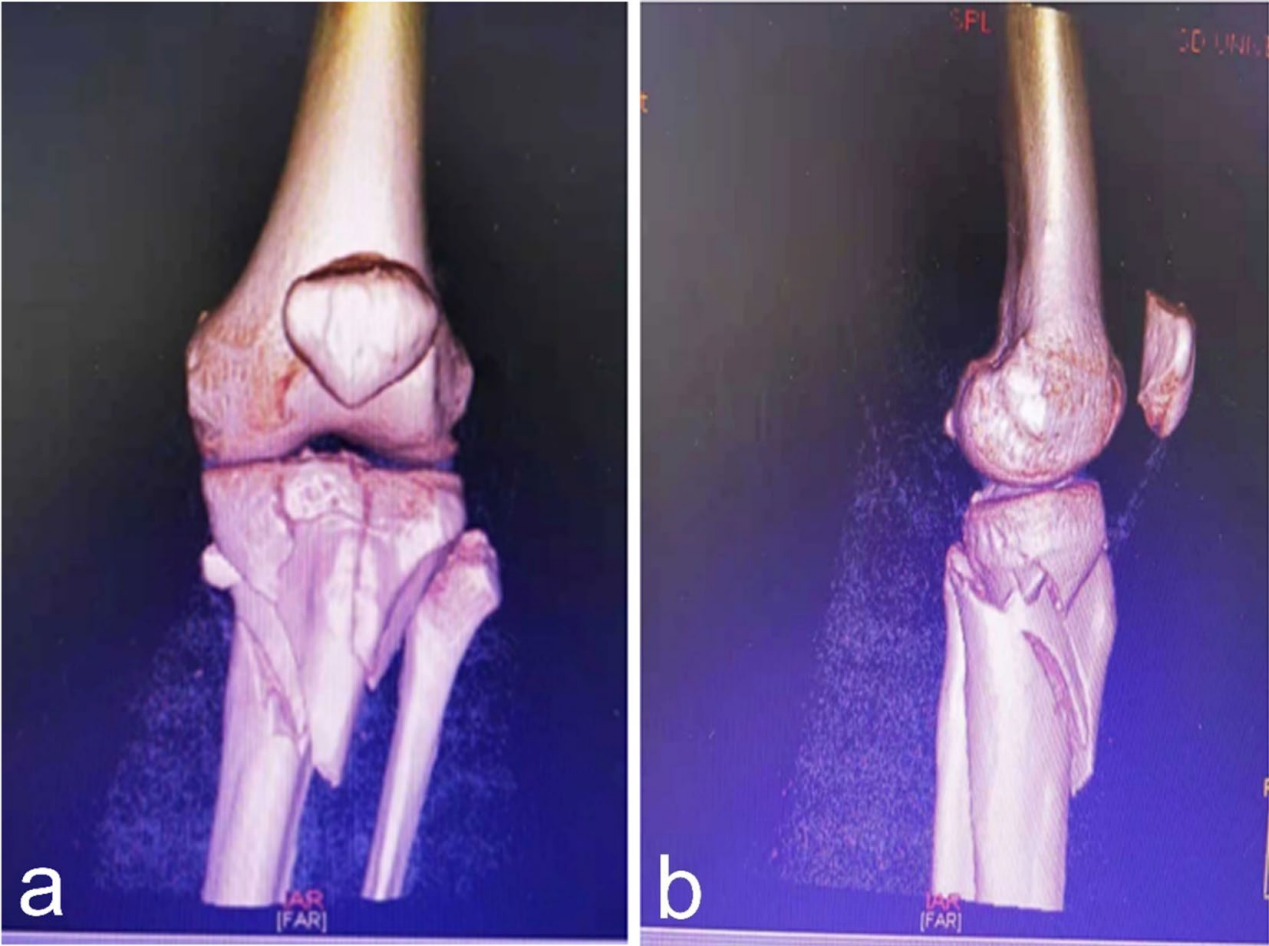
(a,b) CT 3D reconstruction scan for preoperative planning.
